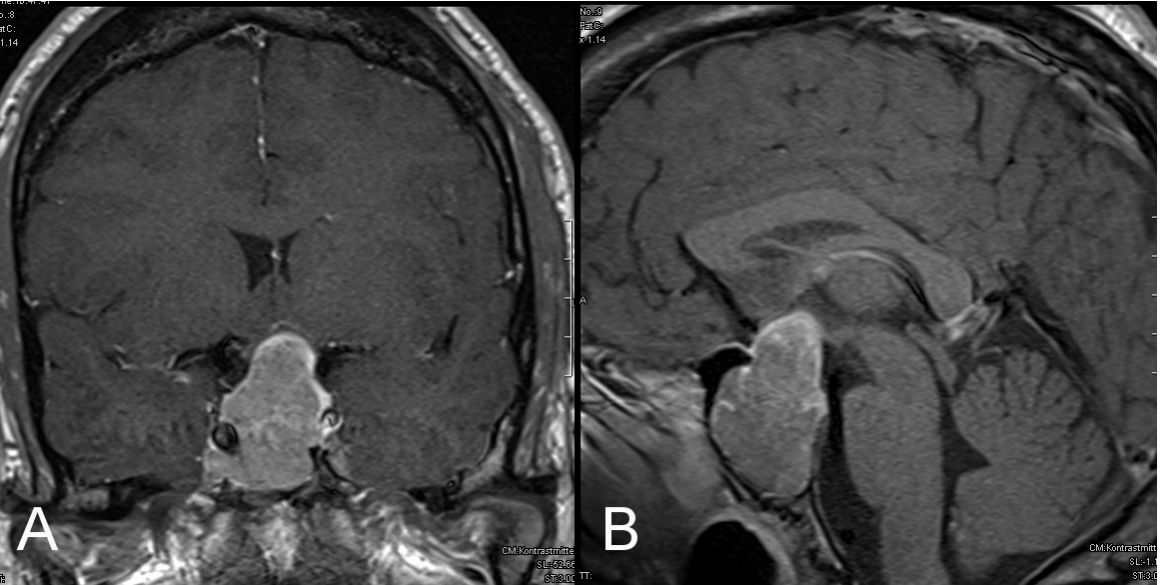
MRIs of tumour site. 5a. coronal 5b. sagittal: MRI scans show extensive growth in and above the sella turcica with a total volume of 4.7 × 2.9 × 2.2 cm^3^.
